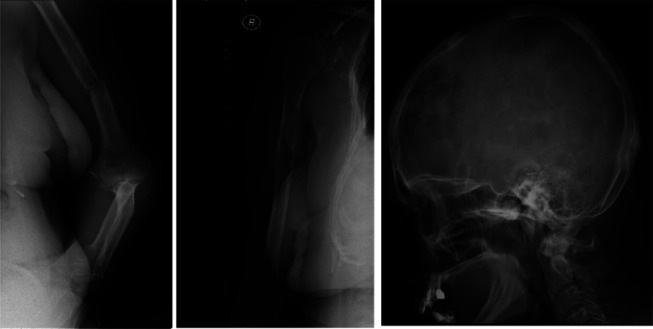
Skeleton X-ray: pathologic bone fracture of the left humerus (left one); multiple osteolytic lesions (middle: right humerus; right: skull).
